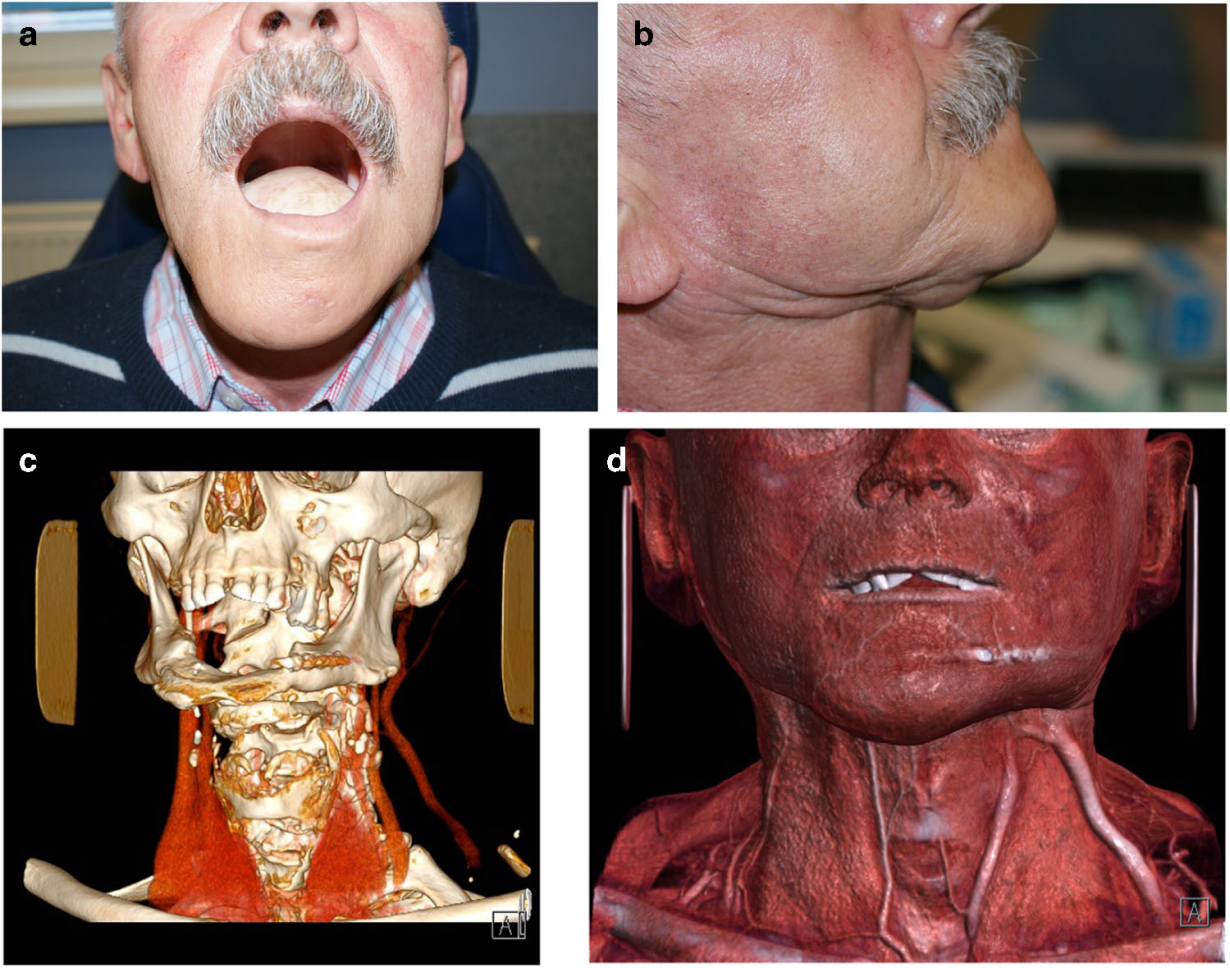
a–d Patient following reconstructive surgery with scapula chimeric flap (comlete removal of the mandibular body and tongue) using 3D model printing. a Frontal view 3 months after surgery with scapula chimeric flap (bone and skin island). b Lateral view 3 months after surgery with scapula chimeric flap (bone and skin island). c CT scan 3 months after surgery with scapula chimeric flap (bone and skin island). d CT reconstruction scan 3 months after surgery with scapula chimeric flap (bone and skin island)
